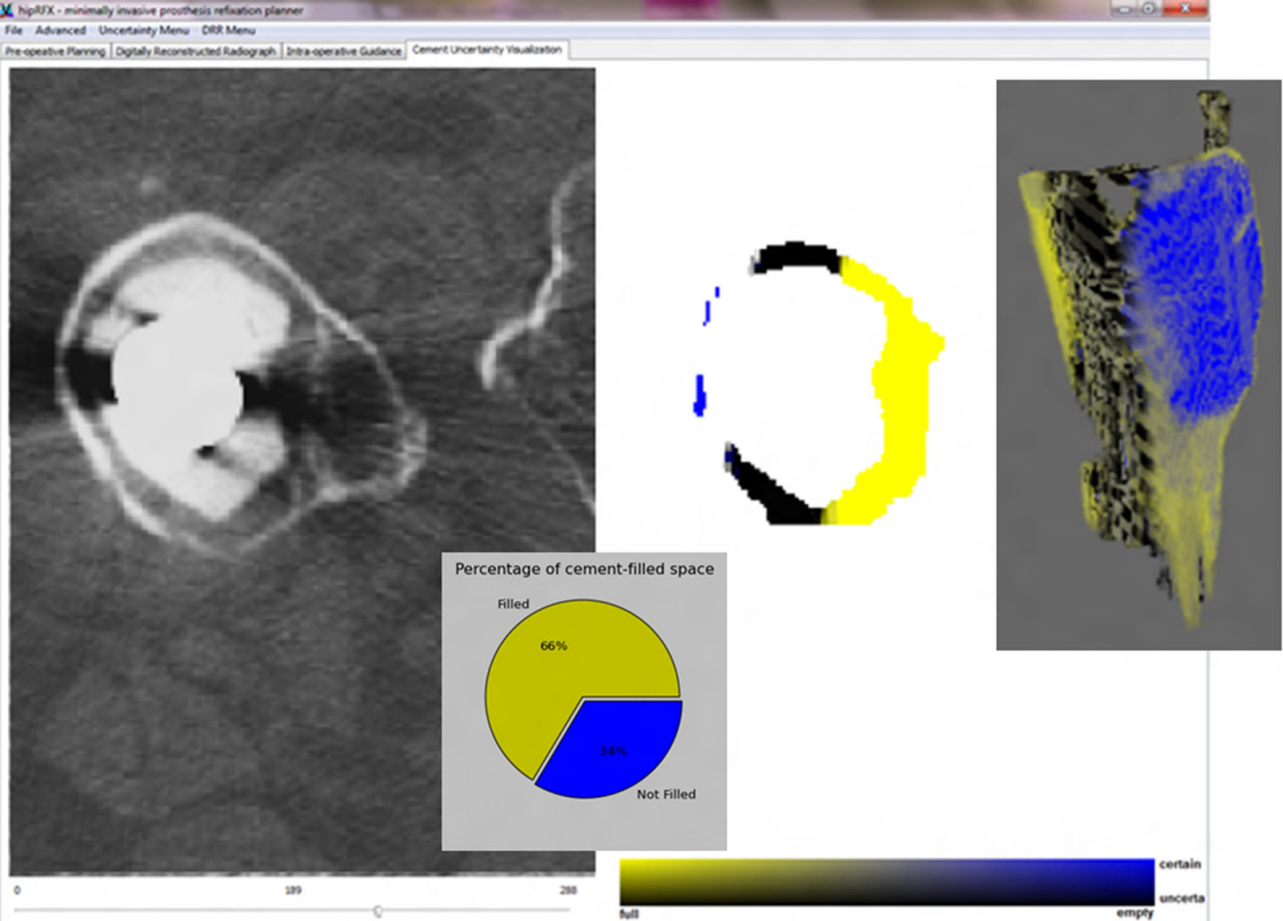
The cement filling feedback panel. A slice through the CT volume is shown on the *left*, with a 3D rendering of the whole cement target on the *right*. *Yellow* indicates target areas that are filled with cement, whereas *blue areas* indicate a lack thereof. Luminance increases with certainty
